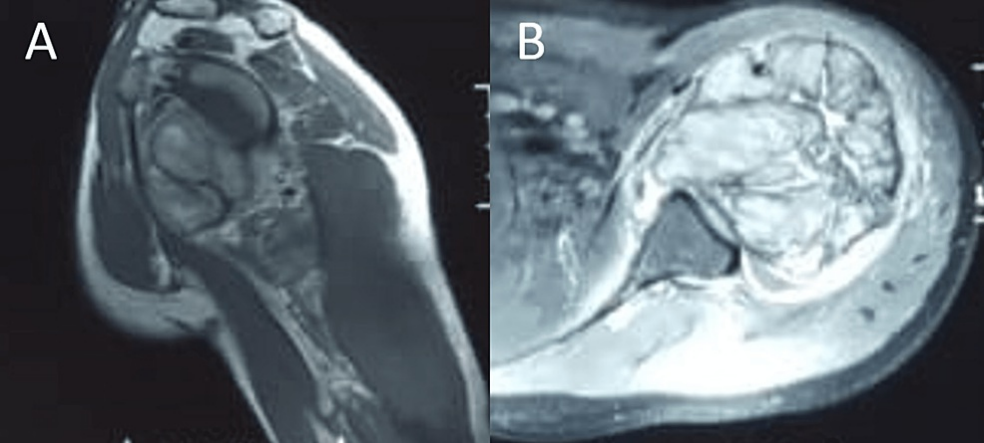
An MRI showed multiple cortical breaches and soft tissue extension; the articular surface was breached with tumor tissue eroding the articular cartilage A: coronal image; B: axial image
